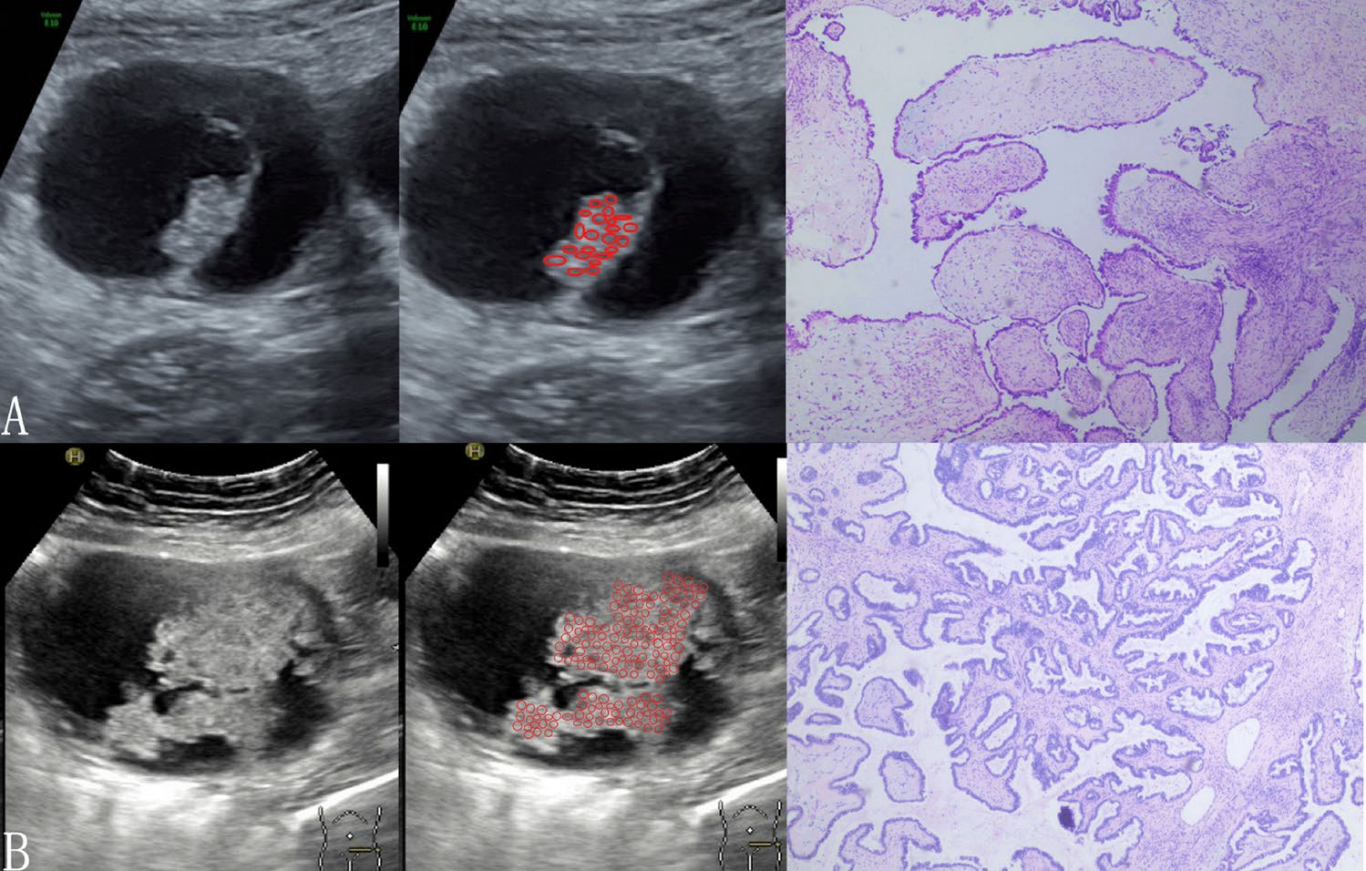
Ultrasound images of ovarian tumors with MCP with pathological controls. The microvesicles in MCP are marked with red circles. On the pathological image, some of the tumors appear as "bubble"-like structures, such as soap bubbles stacked together, with large gaps between the "bubbles" (A). Some of the tumors appear pathologically like a "fork of a tree", with the papillae exhibiting multilevel branching, thin papillae and dense branching, but the tissue gap is still large (B). A Serous borderline tumor; B serous borderline tumor
